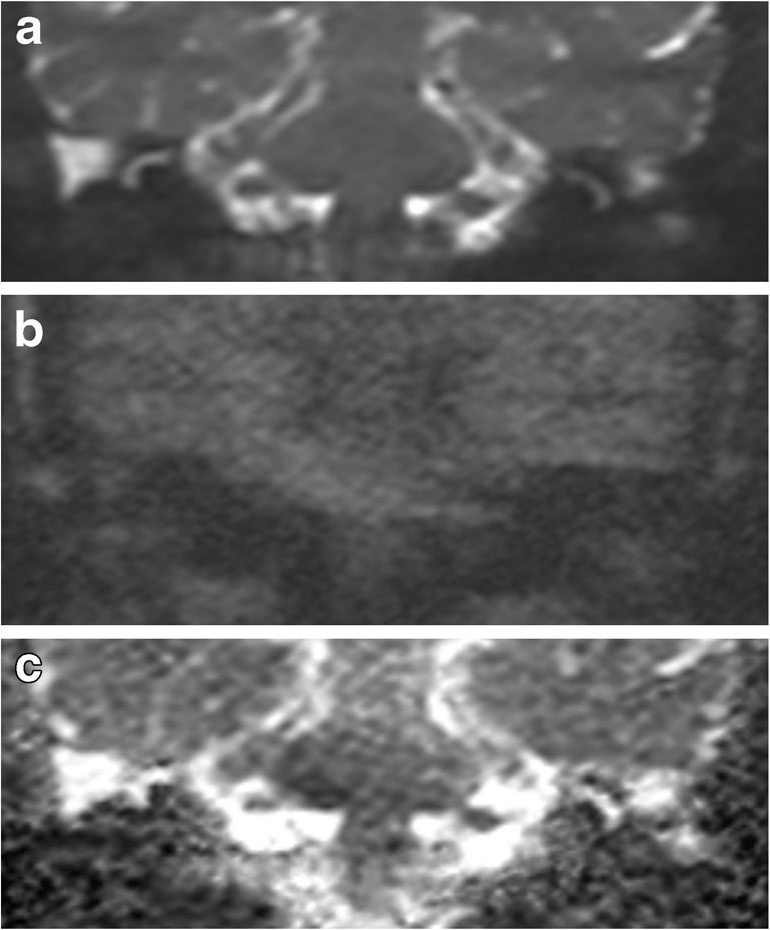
Typical DWI appearance of non-cholesteatomatous soft tissue. Coronal images through the right post-operative mastoid remnant shows the soft tissue (*white arrow*) as high signal on a the b0 image, but loses signal on b the b1000 image, and hence demonstrates high signal on c the ADC map. Surgery confirms granulation tissue
